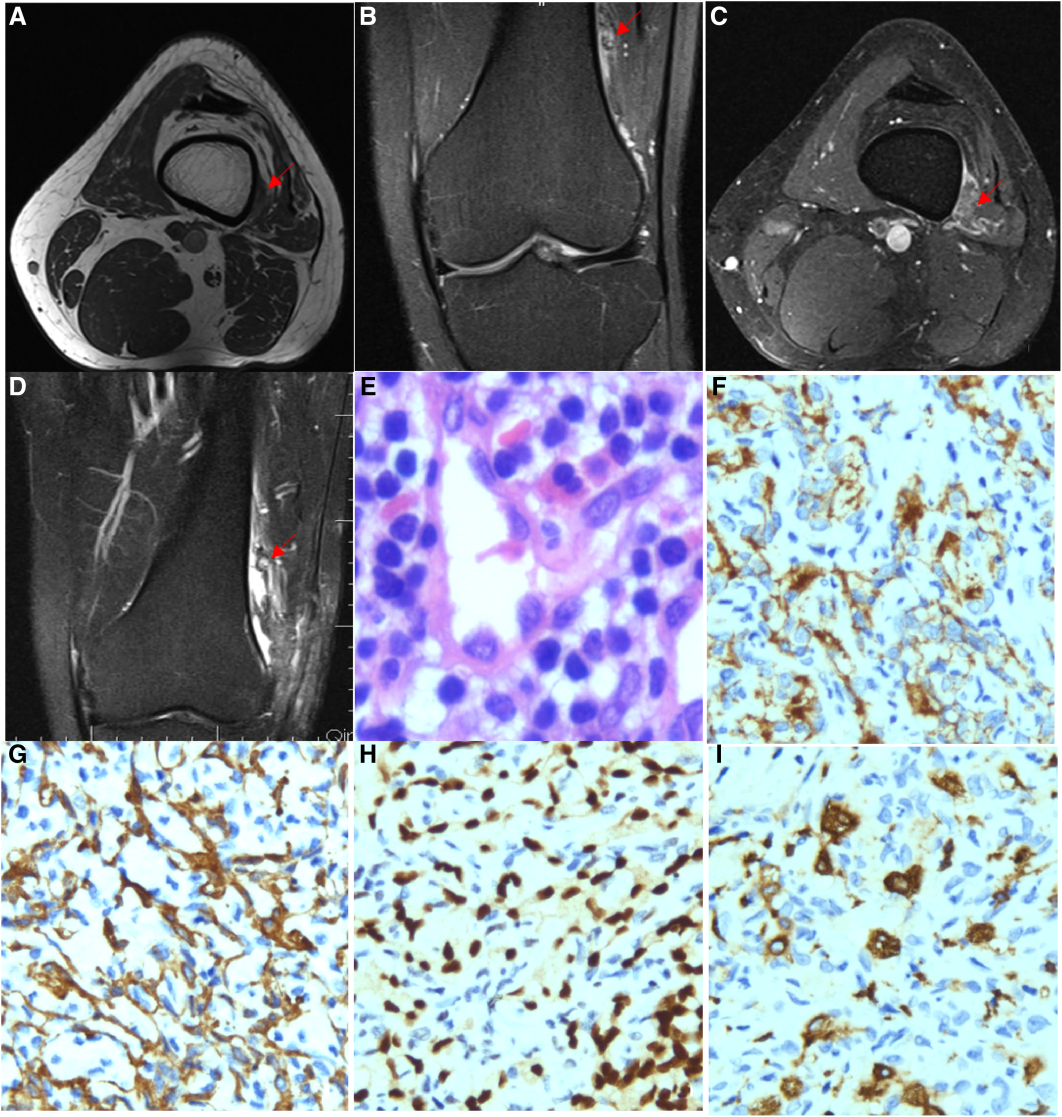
Imaging and pathological features of Case 3. (A) MRI showed a patchy abnormal signal shadow in the vastus lateralis while showing an equal or slightly low signal shadow on T1WI. (B) T2WI-FS showed a high and low mixed signal shadow and a clear edge, and the enhancement scan was slightly enhanced. (C) T2WI-FS series showed a patchy abnormal signal shadow in the vastus lateralis, with a range of approximately 1.5 cm*0.6 cm. No displacement or erosion was observed in the adjacent femur bone, and the bone cortex was intact. (D) T2WI-FS series showed a small nodular residual lesion in the vastus lateralis, with soft tissue oedema and a small amount of fluid in the inner margin. (E) Lymphocyte aggregation (HE ×400), (F) CD31 (IHC ×200) is expressed in endothelial tumour cells. (G) SMA (IHC ×200) is expressed in endothelial tumour cells. (H) ERG is expressed in endothelial tumour cells (IHC ×200). (I) CD163 is expressed in histiocytes (IHC ×200).
